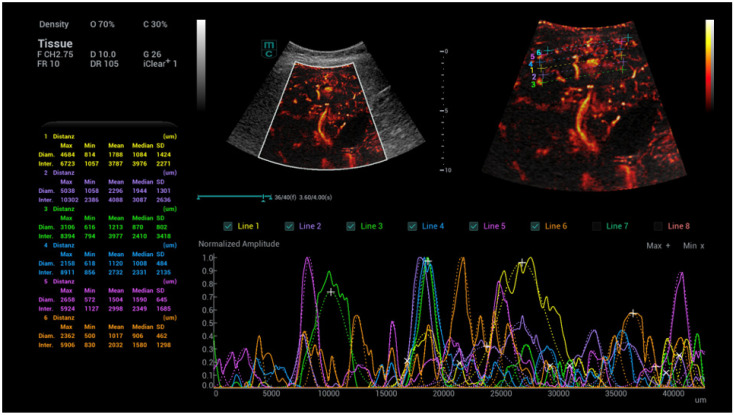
SRCEUS perfusion evaluation in another case after injection of 1.4 mL ultrasound contrast agent with irregular hyperenhancement of the liver and early hyperenhancement of capsular micro-shunts. In the early arterial phase, there is strong contrast enhancement in the areas of micro-shunts with capillary changes of the vascular diameters (line 1) near the capsular structures in comparison to the central vascular structures (line 5).
